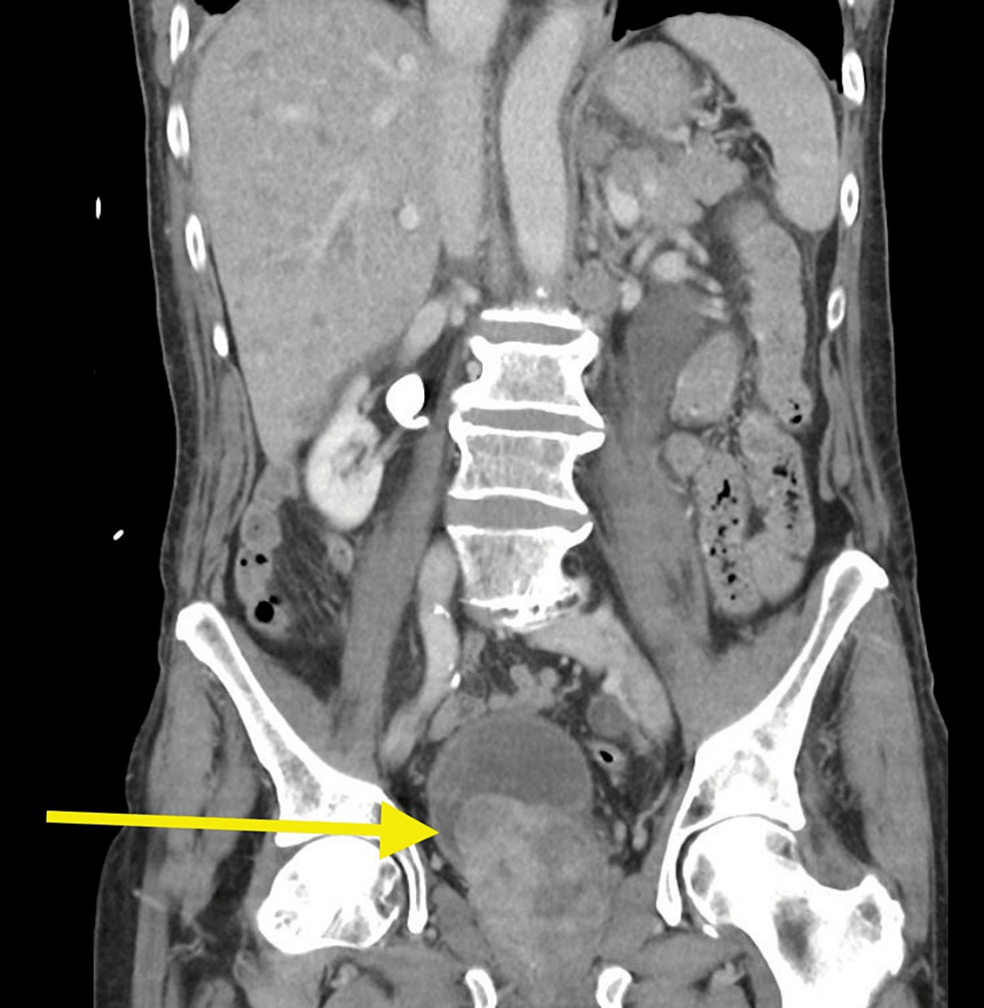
Coronal CT images of the patient’s polypous bladder mass and likely primary site of malignancy CT = computed tomography
